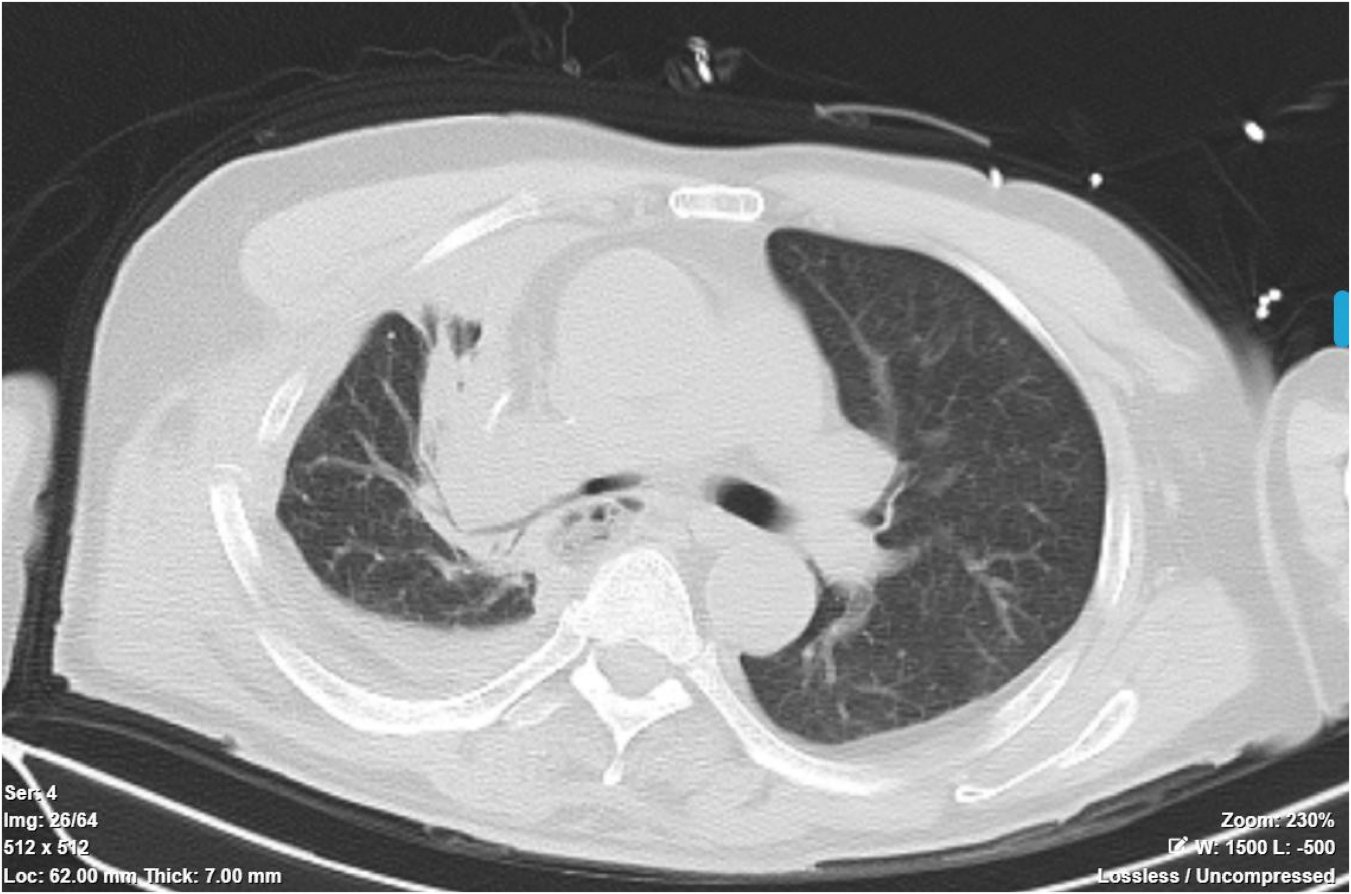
Follow-up chest CT showing lung re-expansion and resolution of pneumothorax after bronchial repair.
